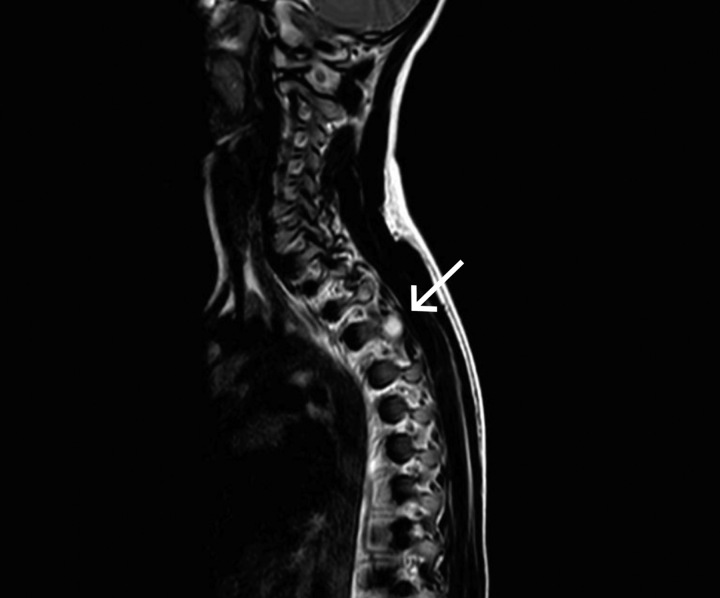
The sagittal T2-weighted MRI image of the thoracic spine reveals a hyperintense lesion measuring 2×2.2×2 cm (arrow).
